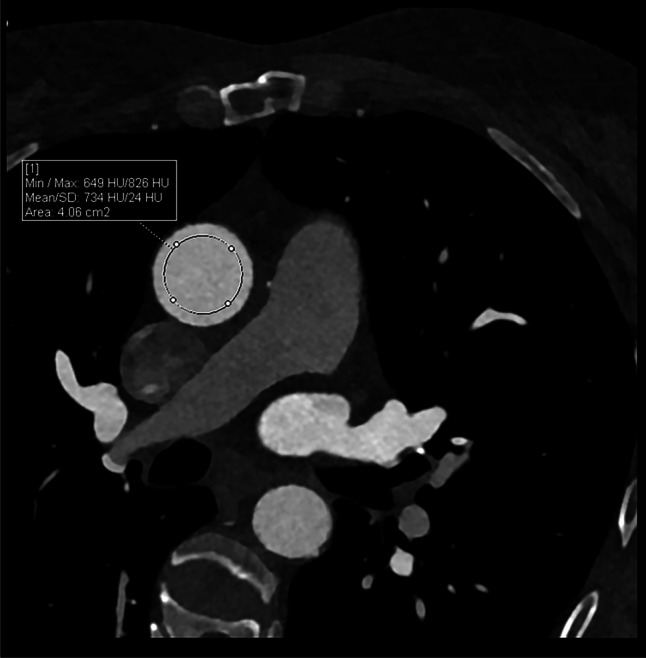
Iodine attenuation measurement. A region-of-interest was placed in the ascending aorta. CT numbers of the region-of-interest together with the standard deviation (noise) were automatically measured
